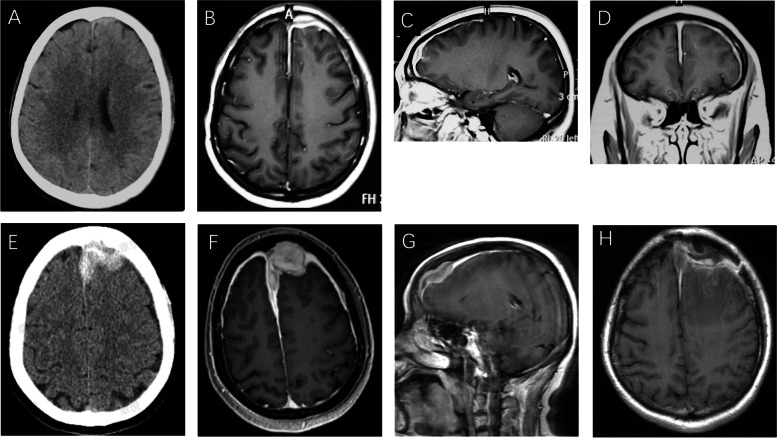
Neuroimages of LPRM. Brain CT in 2016 (A) showed left frontal dural hyperdensity. Axial (B), sagittal (C) and coronal (D) post contrast T1WI showed diffuse dural enhancement involving both convexity and falx cerebri. Brain CT in 2020 (E) showed left frontal dural hyperdense mass. Axial (F) and sagittal (G) post contrast T1WI showed significant enlargement of left frontal lesion and more diffuse dural enhancement than that in 2016. After corticotherapy MRI showed significant resolution of the dural enhancement. (H)
